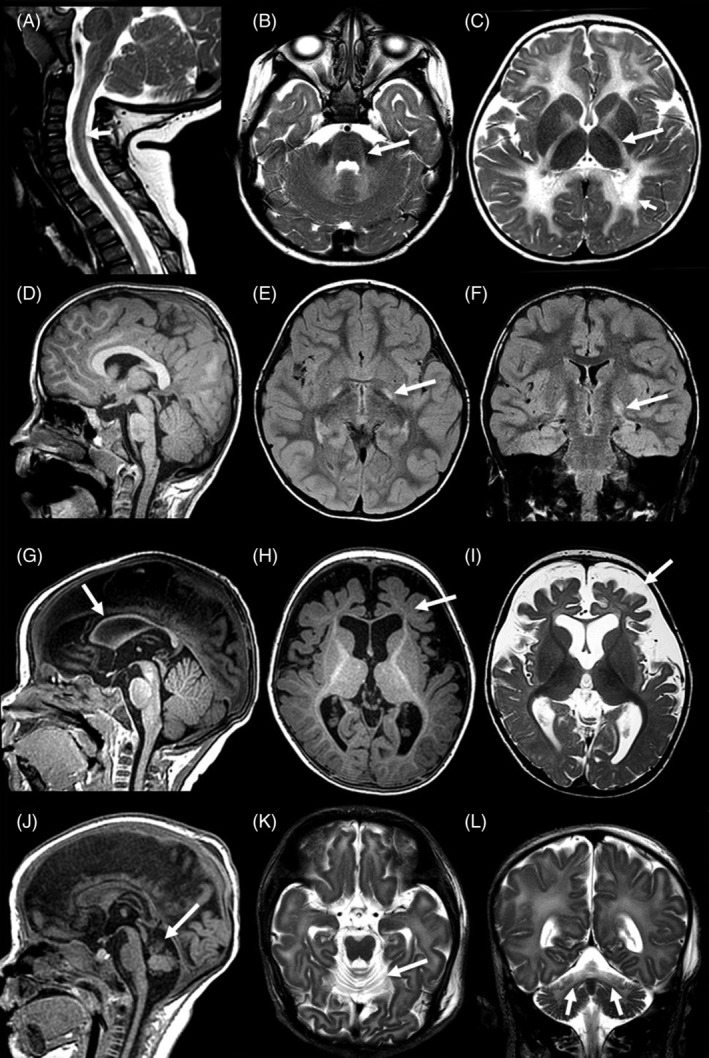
Brain MRI images of four patients with mitochondrial aminoacyl‐tRNA synthetase deficiencies were depicted. A,B,C, Brain MRI of the patient (patient 2) with *DARS2* deficiency at the age of 56 week. T2 weighted sagittal image, A, of the spine showed increased signal intensity in the dorsal column (arrows). T2 weighted axial image, B, demonstrated increased signal intensity in the cerebellar white matter (short arrow) and in the superior cerebellar peduncles white matter (arrow). Axial T2 weighted image, C, revealed involvement of the subcortical and periventricular white matter and the external capsule, anterior and posterior (arrow) limbs of the internal capsules. D,E,F, Brain MRI of the patient with *EARS2* deficiency at the age of 4 years 6 months old. There was bilateral symmetric increased signal in globus pallidus (arrows) on axial, E, and coronal, F, in FLAIR images. G,H,I, Brain MRI of the patient with *PARS2* deficiency at the age of 8 months. Sagittal T1 weighted image, G, demonstrated a markedly thinned corpus callosum. Incomplete myelin maturation of the frontal lobes was shown in axial T1 weighted image, H. Axial T2 weighted image revealed prominent frontal, I, and Sylvian CSF spaces and widened cortical sulci and anterior horns of the lateral ventricles reflecting frontal lobe volume loss. J,K,L, Brain MRI of the patient with *RARS2* deficiency at the age of 6 weeks. Superior vermian atrophy (arrow) was demonstrated in sagittal T1 weighted image. J, Marked superior vermian and superior cerebellar atrophy (arrows) was shown in axial, K, and coronal, L, T2 weighted images
